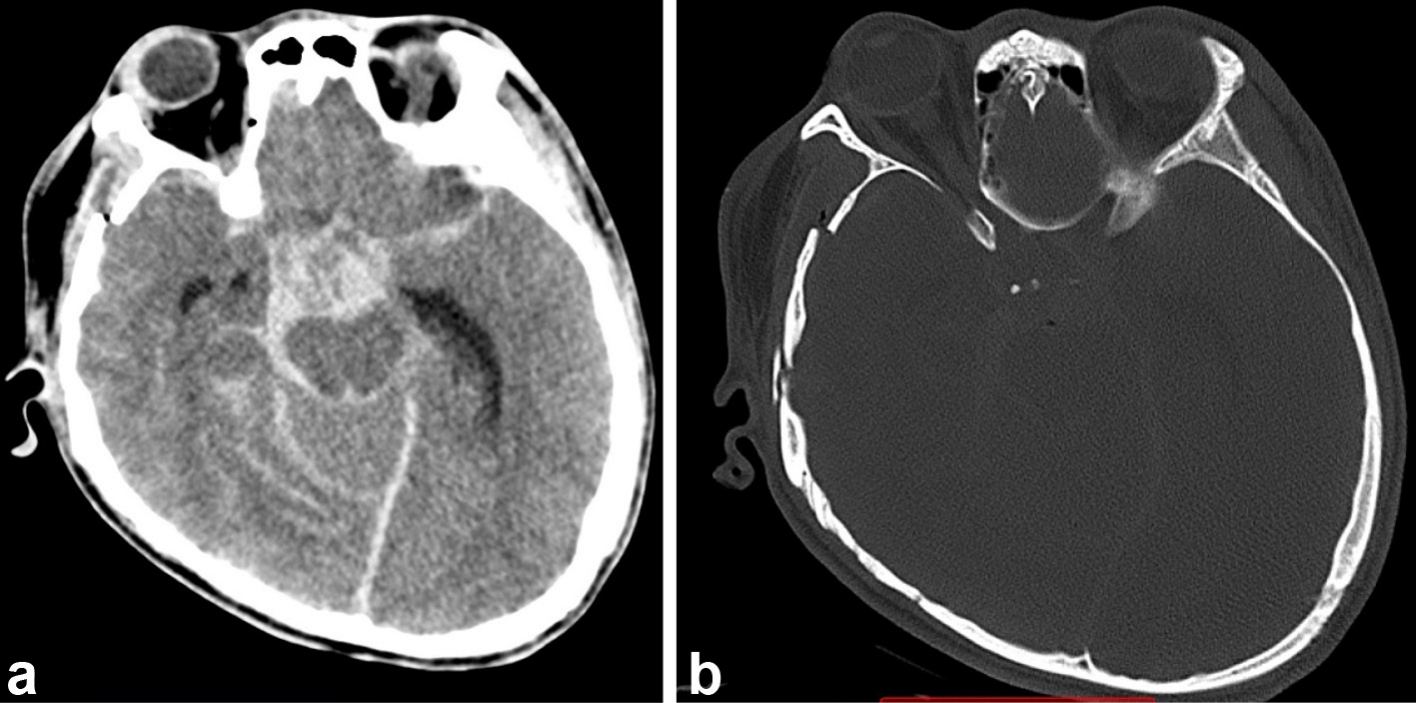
Axial CT images in soft tissue and bone window, at the level of cavernous
